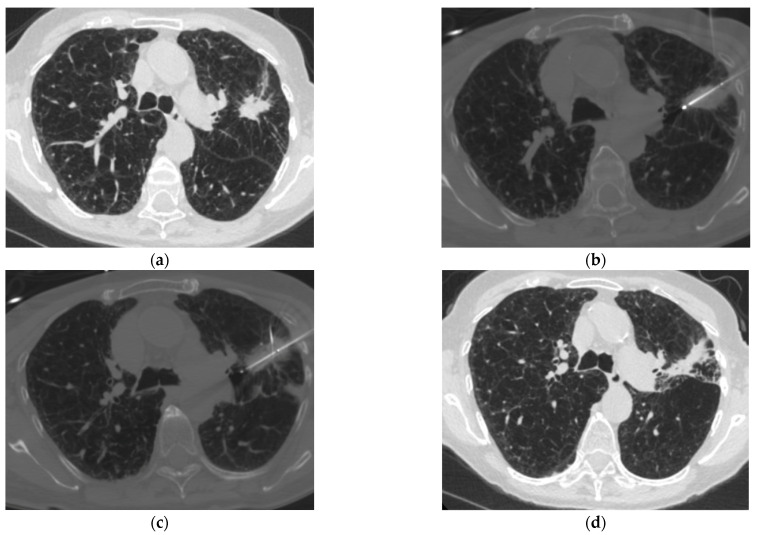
CT-guided percutaneous cryoablation of previously irradiated left upper lobe lung cancer. A 70-year-old female with history of left upper lobe (LUL) cancer status post-radiation presented for cryoablation of enlarging LUL nodule. (a) Pre-operative CT demonstrates moderate-to-severe upper lobe predominant emphysema with biapical pleuroparenchymal scarring and diffuse bronchial wall thickening. A 2.5 × 2.1 cm spiculated LUL lesion represents the recurrence. (b,c) Three cryoprobes were positioned in the lesion and a triple-freeze protocol was performed (3 min freeze, 3 min thaw, 8 min freeze, 5 min thaw, 8 min freeze). (d) A 3-month post-ablation CT shows increased consolidation and volume loss in the treated LUL, consistent with expected post-cryoablation changes.
